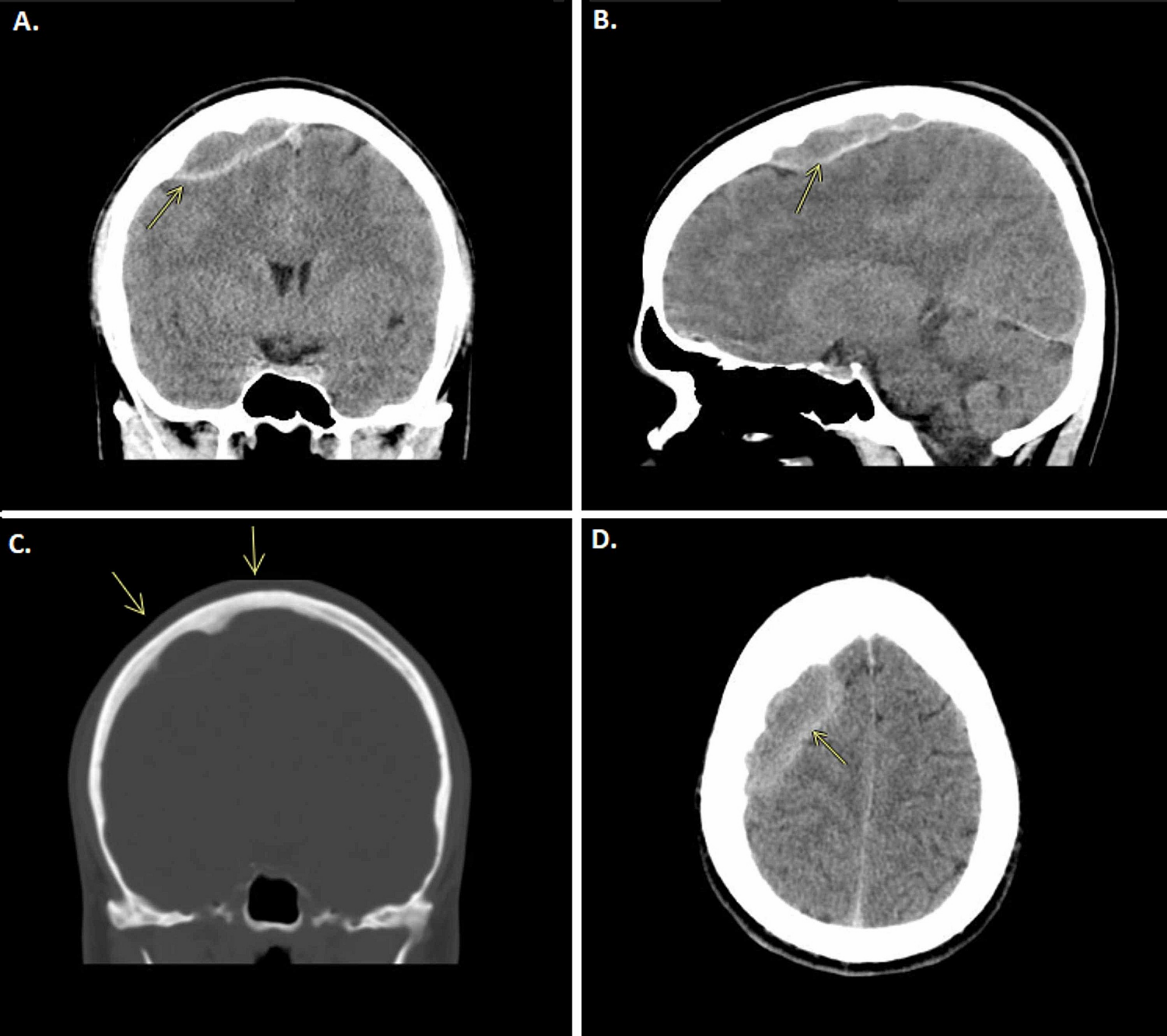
CT scan without contrast of the brain in coronal, sagittal and axial plane. AB, D; CT without contrast, showing the epidural collection of the right frontoparietal area, see arrows. C; CT with bone window, arrows pointing at scalloping in the adjacent neurocranium and suspected osteomyelitis.
